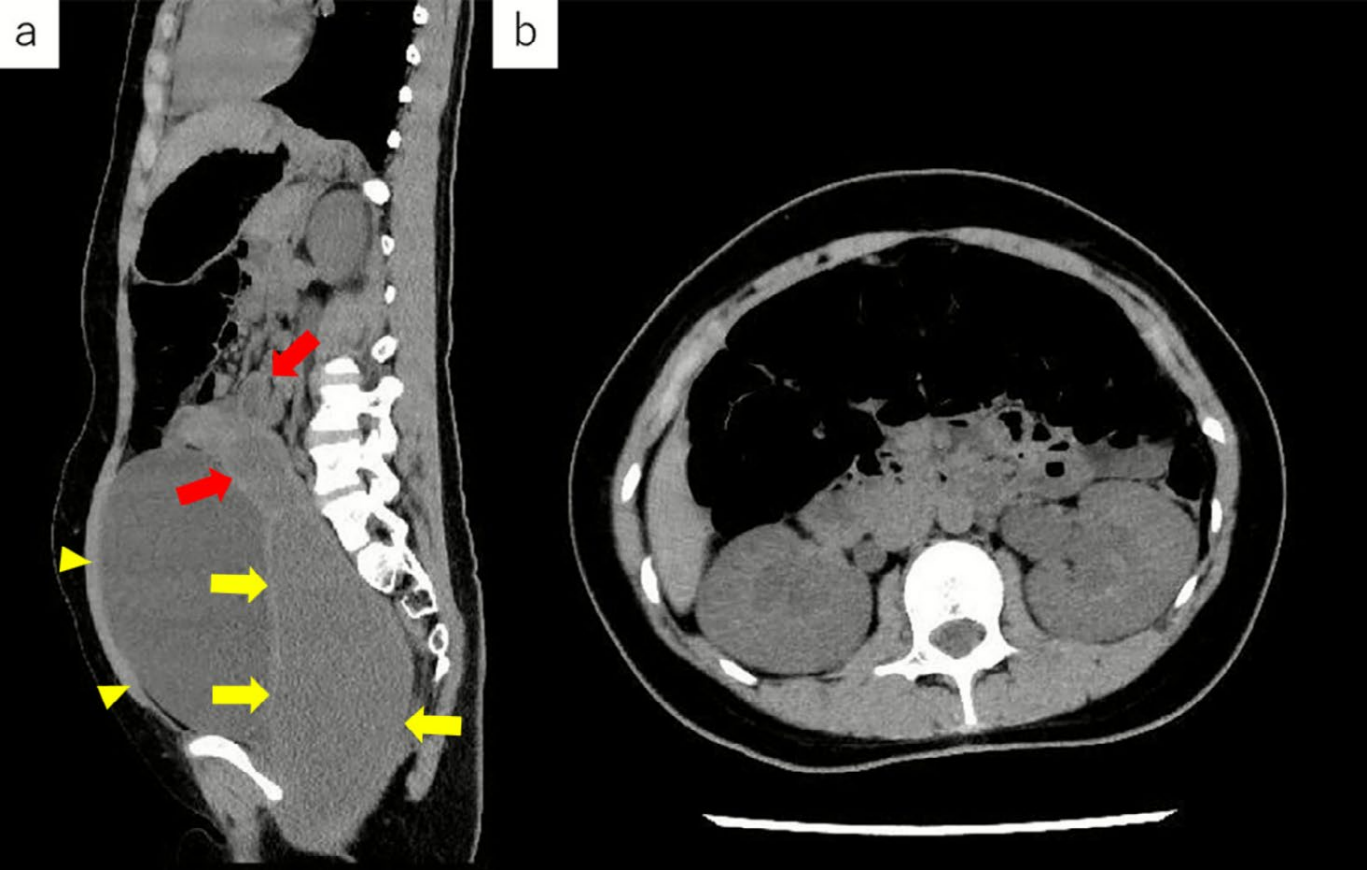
(2a) Sagittal CT demonstrated significant fluid accumulation in the vaginal canal (yellow arrows) extending into the uterine cavity (red arrows), along with pelvic organ prolapse and bladder distension (yellow arrowheads). (2b) Axial CT demonstrated bilateral hydronephrosis and ureteral dilatation.
